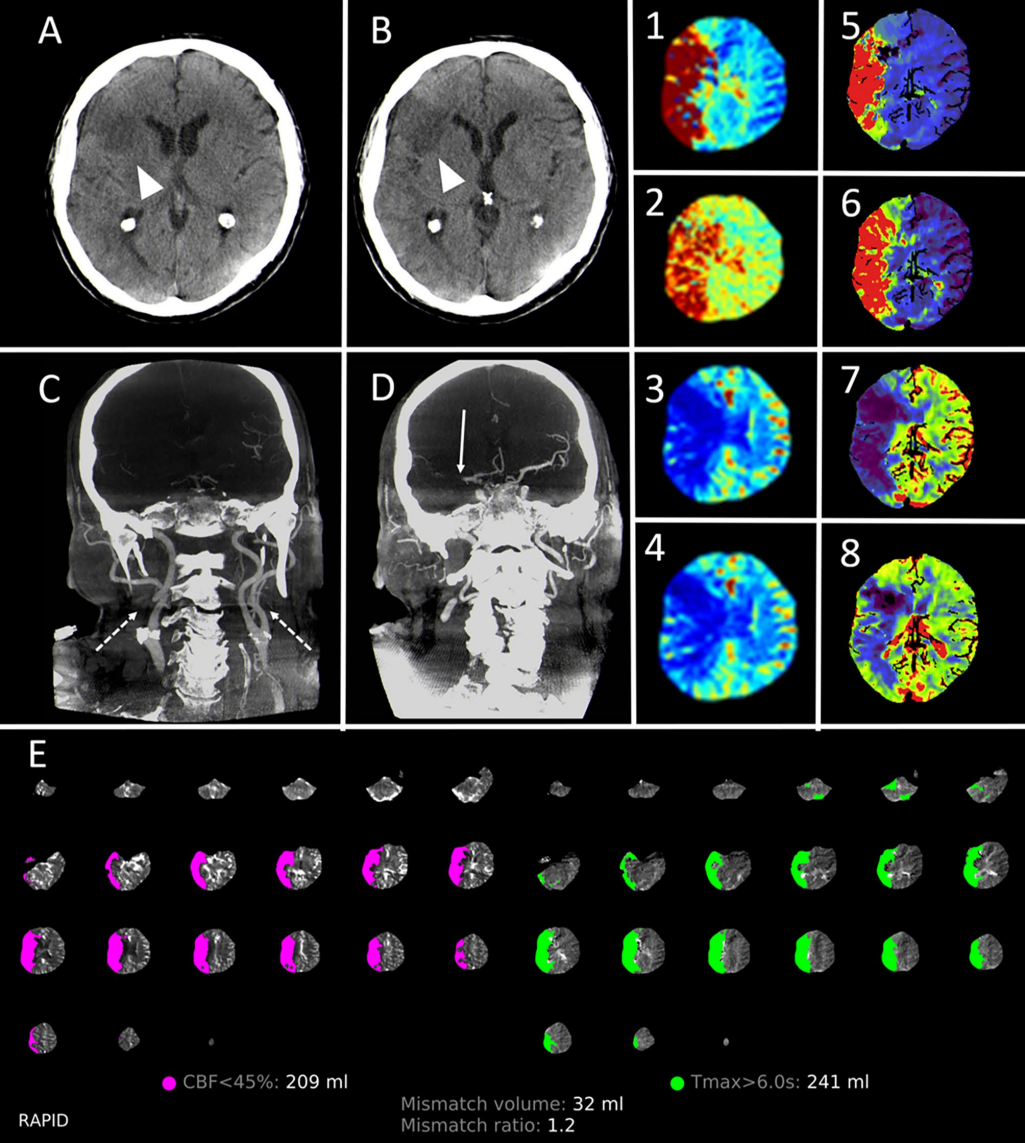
Exemplary images processed by the new multimodal FD-CT approach. 66-year-old male with a proximal M1 occlusion right, NIHSS 21. NE-FDCT on which the demarked infarct of the anterior third of the right MCA-territory can be delineated (A and B; arrowheads). FD-CTA shows a clear visualization of the cervical vasculature (C; dashed arrows) and a proximal occlusion of the right M1-segment (D; continuous arrow). FD-CTP shows a matching deficit in the color-coded maps of RAPID ANGIO (1–4) and of the Siemens perfusion prototype software (5–8) as they show a delay for Tmax (1) and TTP (5), MTT (2 and 6) and a reduction for CBF (3 and 7) and CBV (4 and 8). Automated perfusion analysis using RAPID ANGIO revealed a small mismatch between the infarct core, estimated by the CBF reduction (pink), and the potential salvageable tissue, estimated by the delayed Tmax (green), which results into a mismatch ratio of 1.2 (E)
